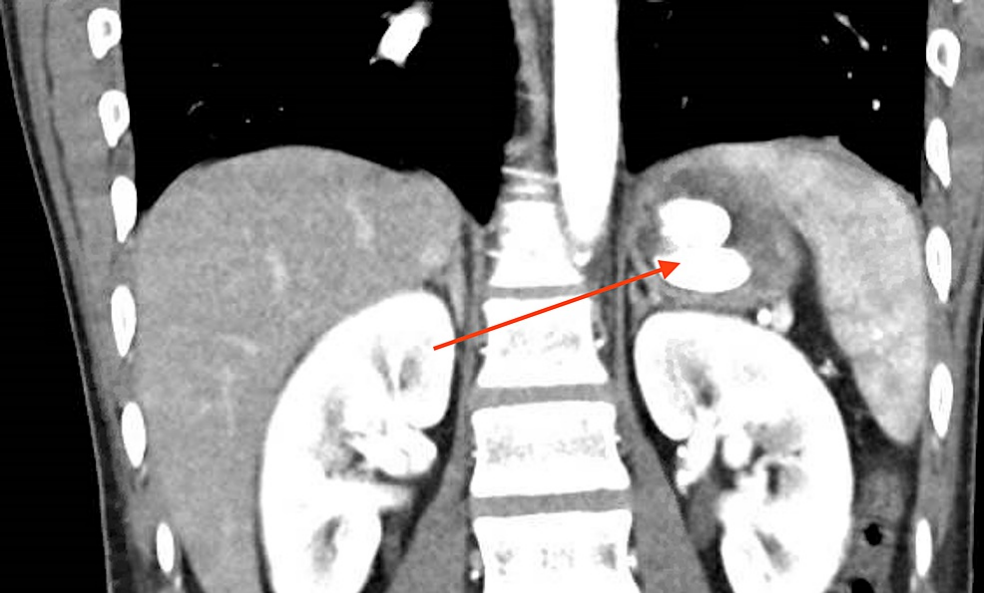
Two foreign bodies seen in the stomach on CT scan with red arrow indicating location CT, computed tomography
